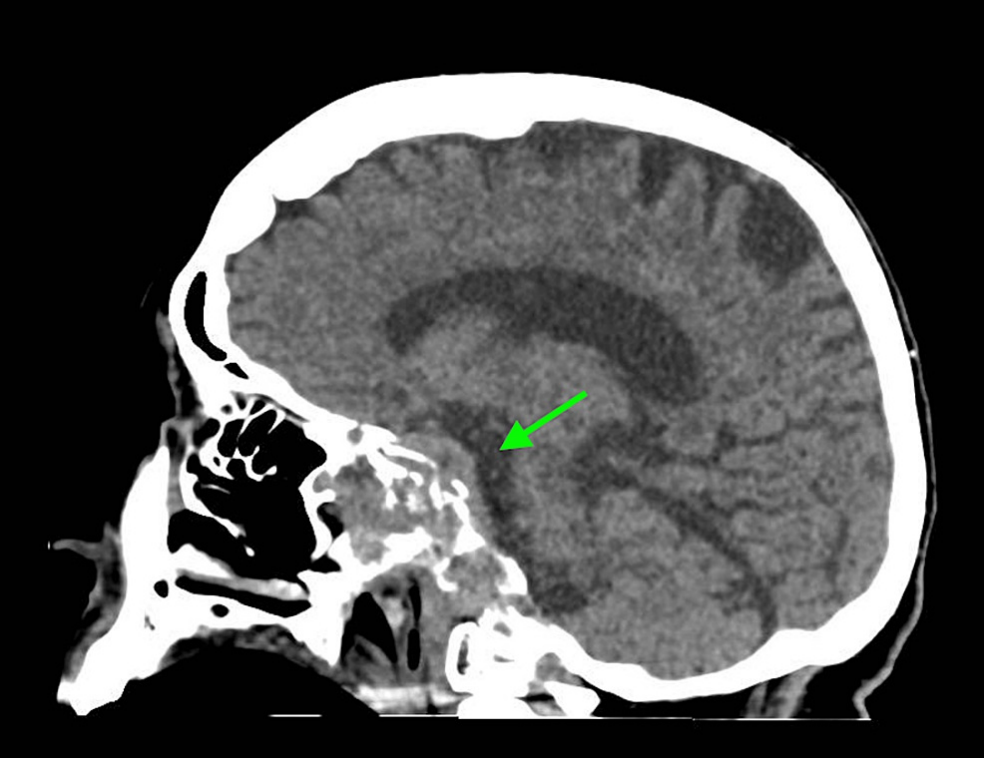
Sagittal image obtained from computed tomography of the head demonstrates clival chordoma with sphenoid sinus obliteration, involvement of the cavernous sinus, and bilateral petrous apices (green arrow)
